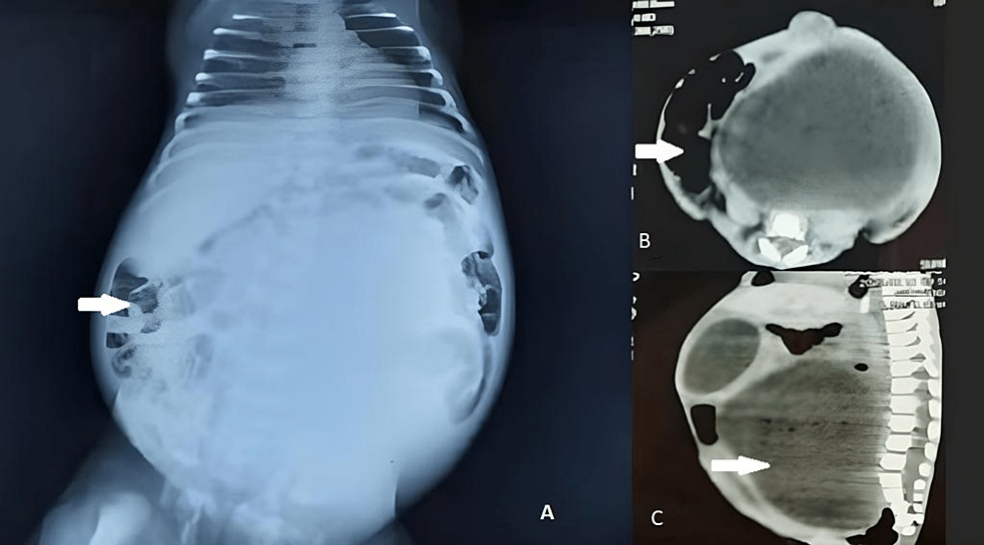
(A) X-ray of the abdomen. (B, C) CT scan showing hydrometrocolpos.
